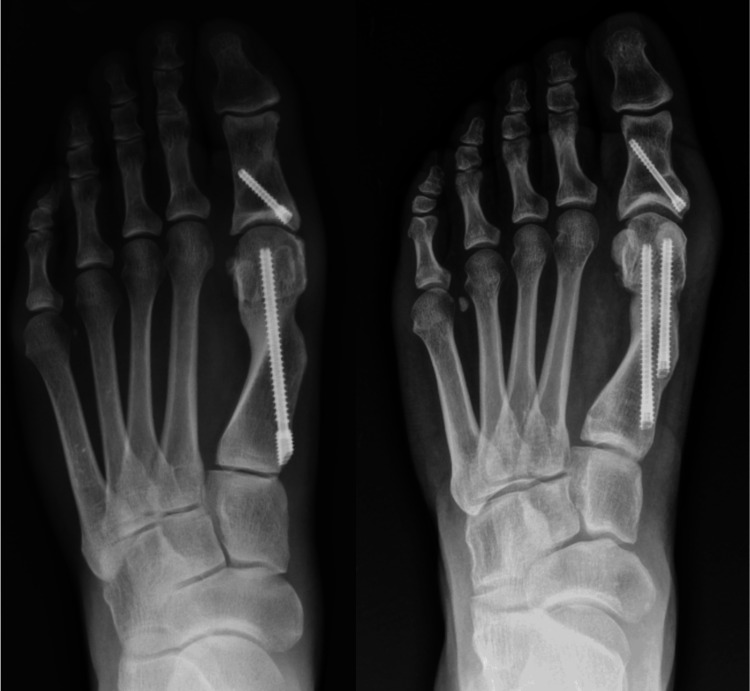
Radiographs demonstrating single-screw (left) and dual-screw (right) fixation following minimally invasive distal metatarsal osteotomy for hallux valgus correction. Radiographs courtesy of Dr. Ettore Vulcano and Dr. Peter Lam.
